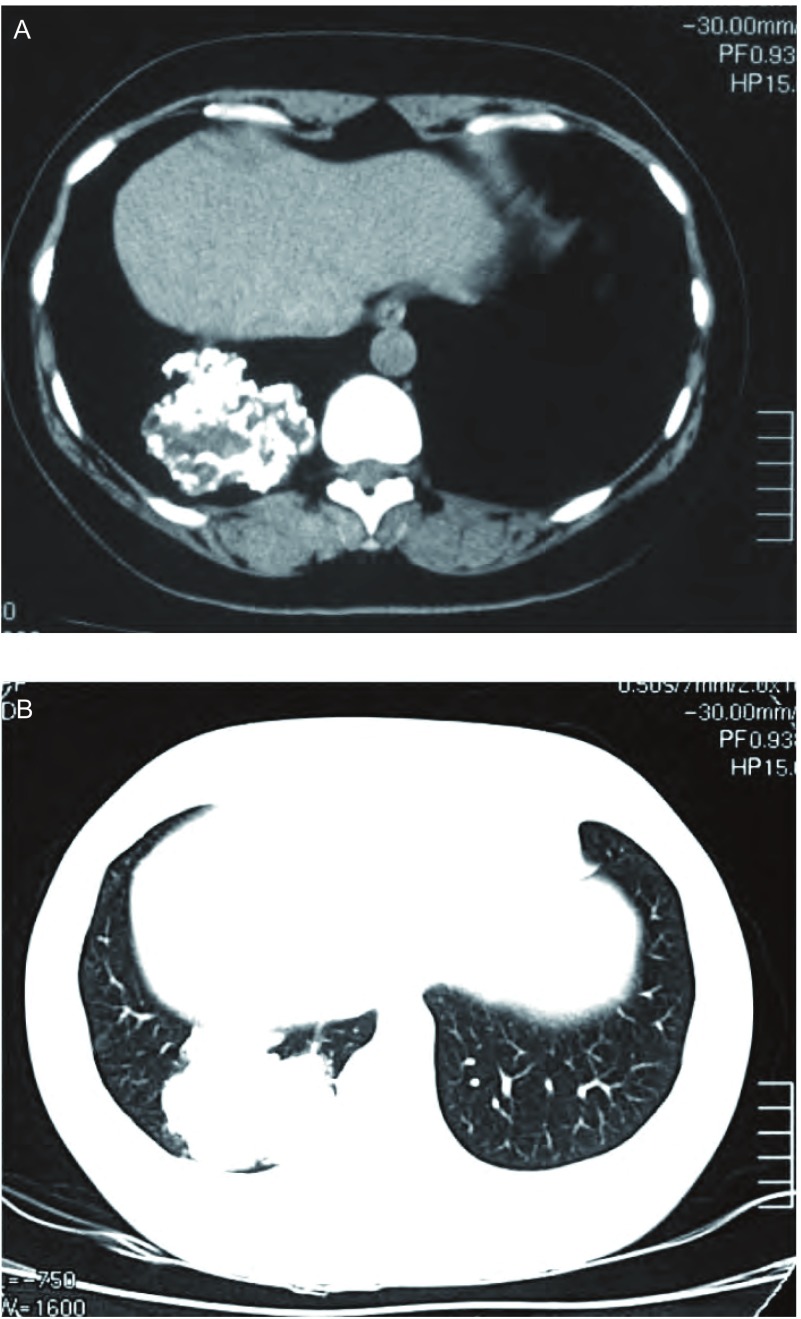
胸部CT检查。A：纵隔窗显示右下肺软组织肿物，内见多发钙化；B：肺窗显示肿物位于右下肺内。 CT scan of the chest. A: in mediastinal window, the tumor was located in the right lower lobe, multiple calcifications were existed in the tumor; B: in lung window, the tumor is in the parenchyma of right lower lobe.
